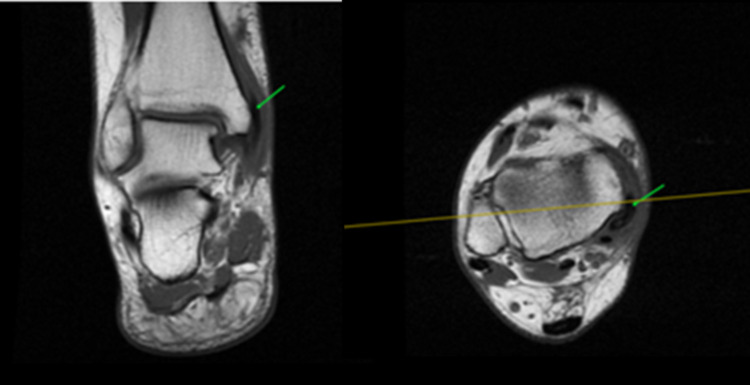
Ankle MRI-T1 sequence (coronal view-left, axial view-right) showcasing transposition of PTT (green arrow) over the medial malleolus. Yellow line indicating same anatomical level in axial compared to coronal plan. MRI: Magnetic Resonance Imaging PTT: Posterior Tibialis Tendon
